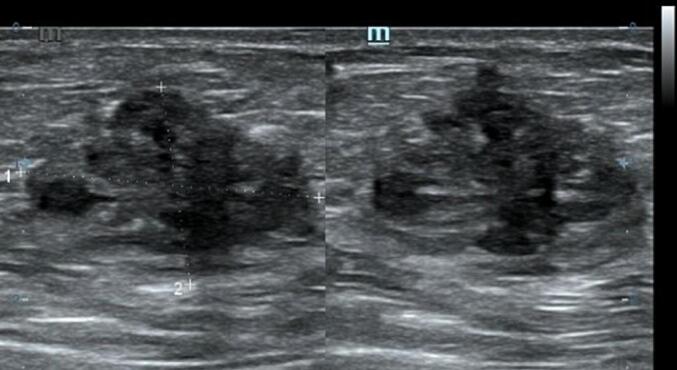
Ultrasonography examination of the first patient showed a heterogenous hypoechoic lesion in the subcutaneous region of the lower abdomen suggestive of endometriosis.
